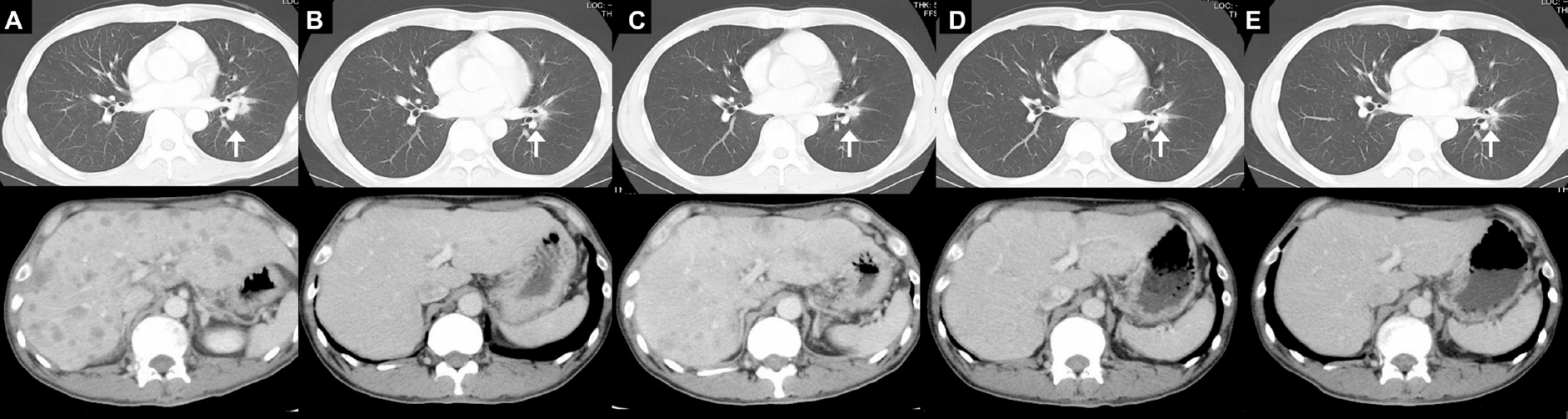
Contrast-enhanced CT scans of the lungs and liver Images were acquired before treatment with gefitinib (A), 11 days after the onset of gefitinib treatment (B), 7 months after treatment onset, when disease progression was apparent for the liver metastases (C), 1 month after the onset of treatment with osimertinib (D), and after 3 months of treatment with osimertinib, when leptomeningeal metastasis had occurred (E). Arrows indicate primary lung lesion.
